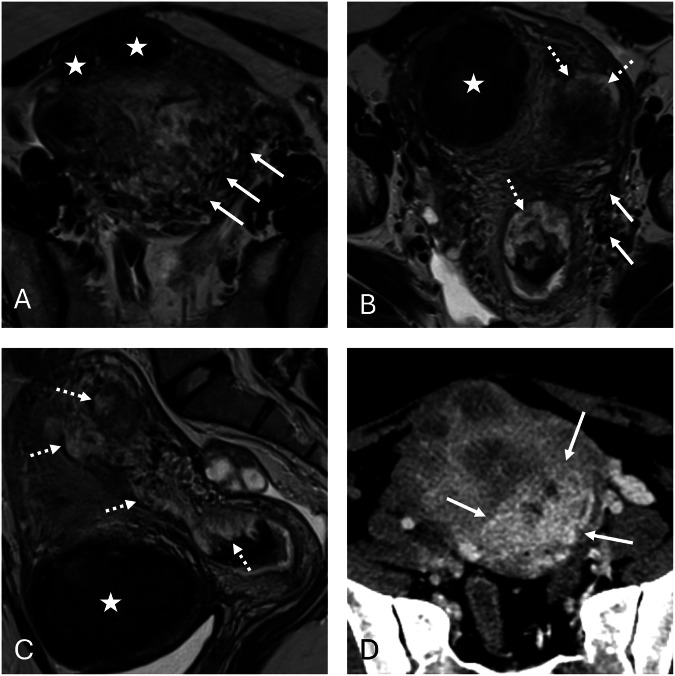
Gestational trophoblastic tumour (choriocarcinoma) in a 27-year-old, G2P2, presenting with postpartum metrorrhagia and elevated HCG. A, B Axial and C sagittal T2-W MR images show a poorly defined T2-hyperintense enlargement of the left corporeo-isthmic region of the uterus, with numerous flow-voids (arrows) and intracavitary tissue components invading the myometrium (dotted arrows). A vaginal metastasis was also visible (not shown). Incidental uterine fibroids are also present (stars). D Axial post-contrast CT image at the arterial phase shows early enhancement of a myometrial tissue infiltration with ill-defined margins (arrows)
